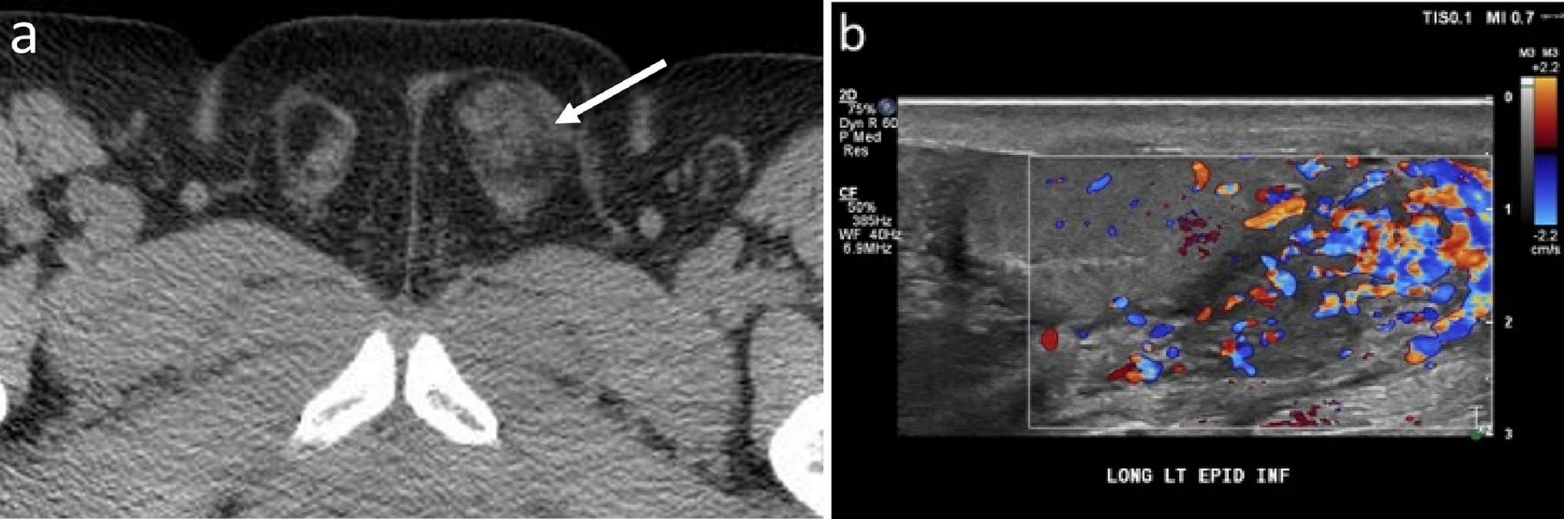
59-year-old patient with hematuria, pyuria and pain radiating into scrotum. All readers reported an abnormal left cord with cord enlargement and inflammatory change visible at NECT (arrow, a). Reader confidence levels were lower, ranging from 1 to 3 on a 5-point Likert scale. Color Doppler ultrasound confirmed left-sided epididymitis (b). Cord abnormalities were not appreciated at the prospective CT read
